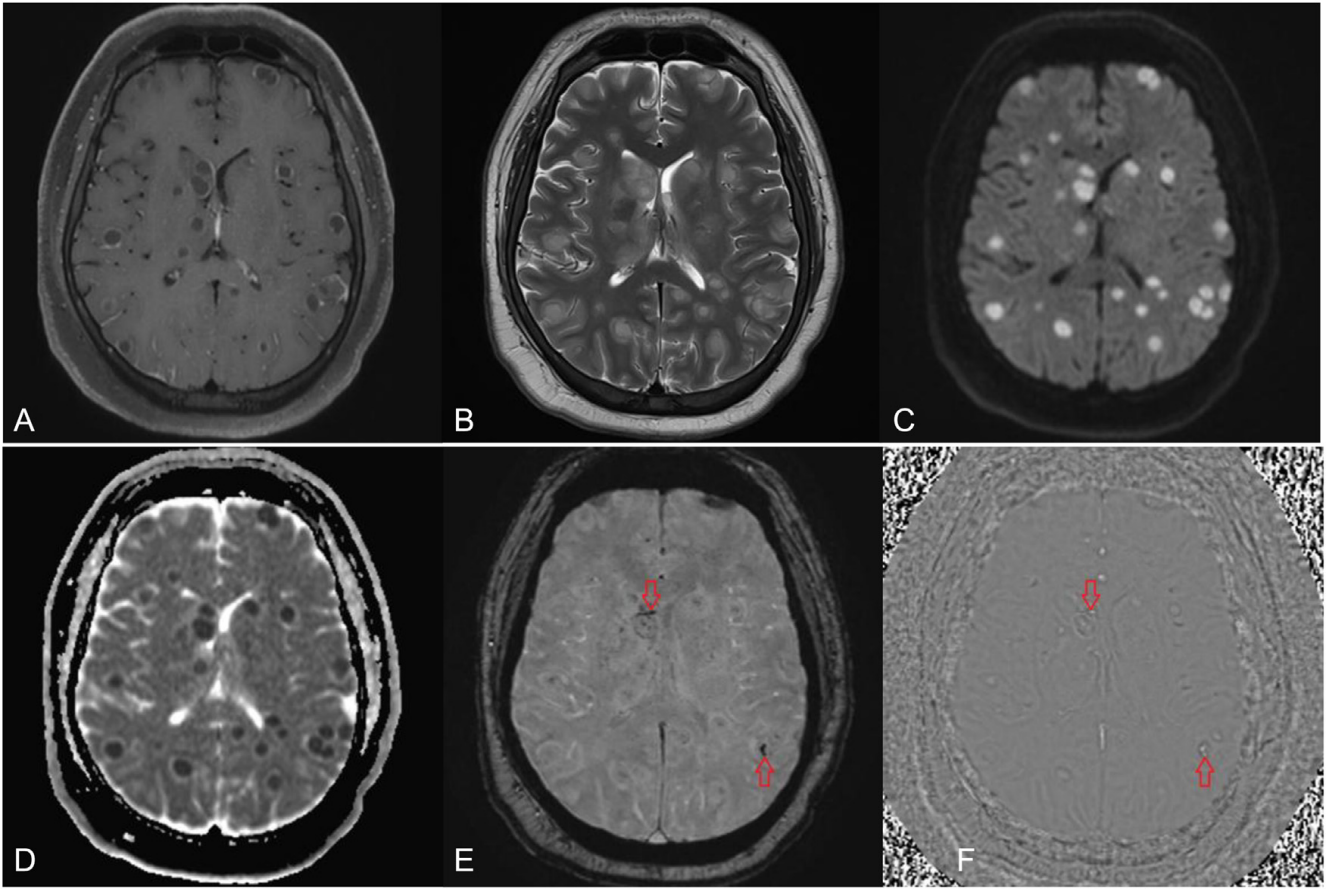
MRI head performed 2 days after presentation with axial postgadolinium contrast T1-weighted turbo spine echo image with fat saturation (A) showing multiple ring enhancing lesions. axial T2-weighted turbo spin echo (B) showing only mild lesional hyperintensity and minimal perilesional edema, axial diffusion-weighted imaging, DWI (C) and apparent diffusion coefficient, ADC map (D) showing profound, uniform restricted diffusion within all lesions (hyperintense on DWI and hypointense on ADC) and axial susceptibility-weighted imaging, SWI (E) and SWI filteredphase image (F); showing hemorrhagic foci (red arrows).
